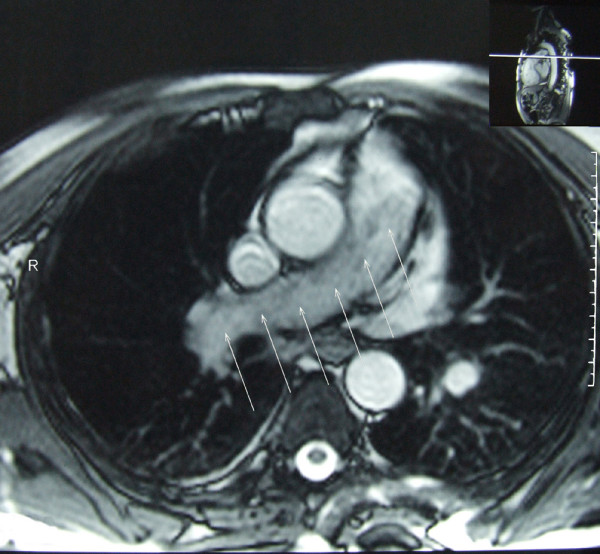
Contrast-enhanced magnetic resonance image of the large blood vessels showing a large filling defect in the pulmonary artery trunk and complete occlusion of the right branch with a discreet increase in signal intensity after contrast injection (arrows).
